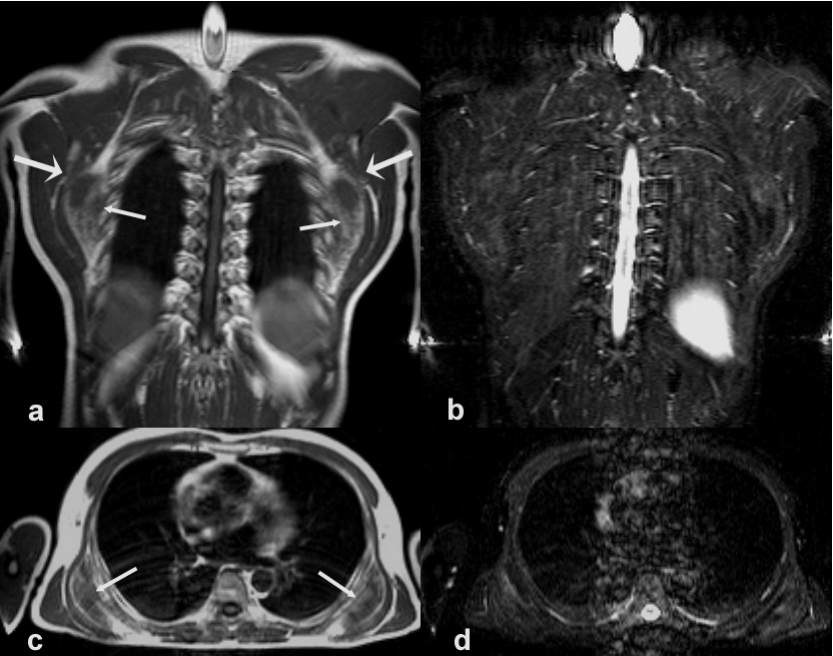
MRI of bilateral elastofibroma with the tumour being located between the thoracic wall, the anterior serratus, and the latissimus dorsi muscle (coronal (2a) and axial (2c) T1-weighted images): The small arrows indicate the medial margins of the lesions containing fatty (bright) and fibrous (dark) tissue. The tumours are located between the thoracic wall, the anterior serratus, and the latissimus dorsi muscle. The large arrows points to the margo inferior of the scapula. Figures [2b] and [2d] show the corresponding STIR -sequences with a slightly inhomogenous signal intensity within the elastofibromas.
